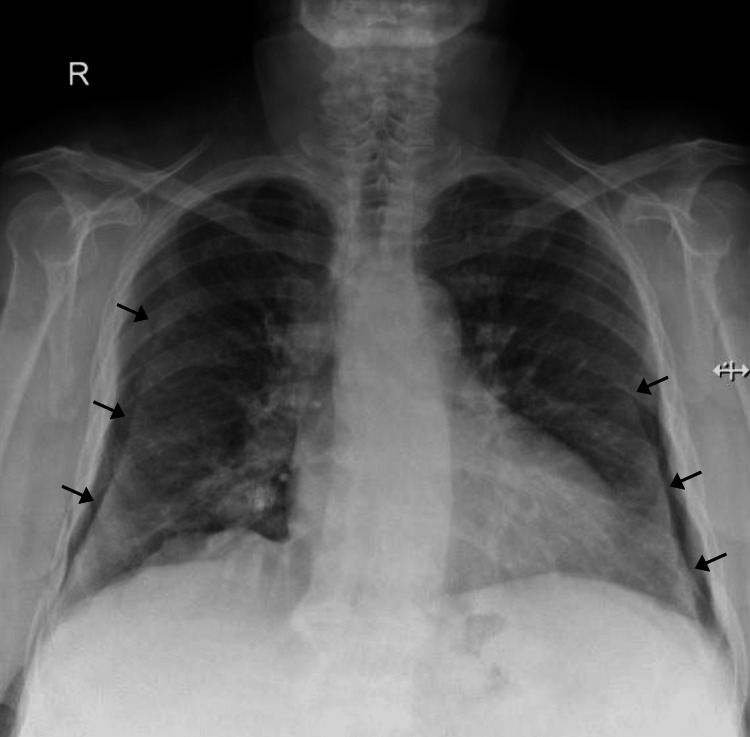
Postero-anterior (PA) chest X-ray showing bilateral curvilinear lines similar to the pleural lines seen in pneumothorax (black arrows).
